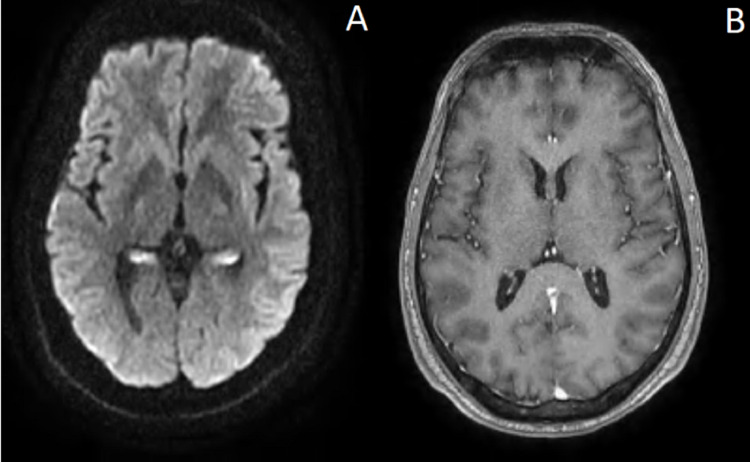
Brain magnetic resonance shows no cerebral abnormality. The cerebral cortex appears to have hyperintensity on diffusion-weighted imaging (DWI). It's an artifact of MRI at our institution. This MRI has been compared to other normal MRI images in our institution and showed no abnormality.
